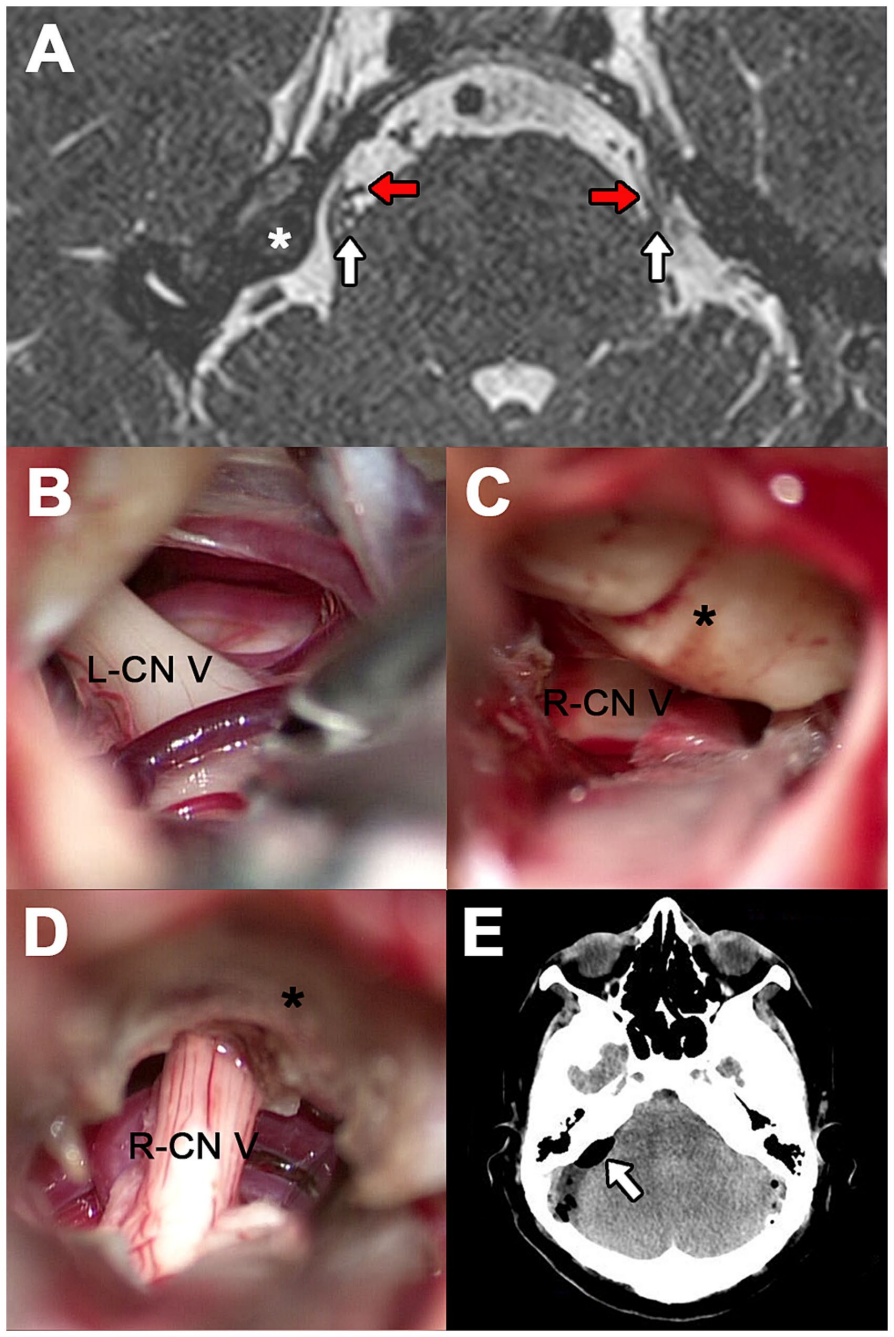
Case 2. (A) 3D-FIESTA MRI showing both CN V at the level of the pons (white arrows). The red arrows indicate the contact vessels and the asterisk shows a lobulated osseous prominence of the right petrosal surface. (B) SCA and a circumflex vein over the left CN V. (C) Prominent suprameatal tubercle (asterisk) obstructing the clear visualization of the CN V. (D) Redundant AICA compressing the CN V revealed after drilling the suprameatal tubercle (asterisk). (E) Postoperative cranial CT scan. The arrow indicates the pneumocephalus in the right anterolateral cistern of the brainstem.
